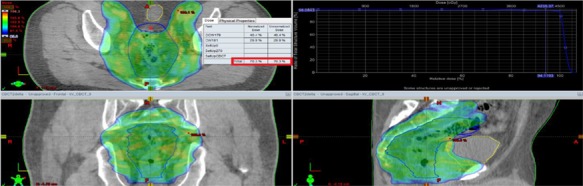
Re-planning with VMAT on cone-beam CT and dose distribution for the mesorectal volume. Mesorectum contour has moved beyond the planned 95% isodose line on CB-CT with an up to 30% dose decrease according to planned dose.
